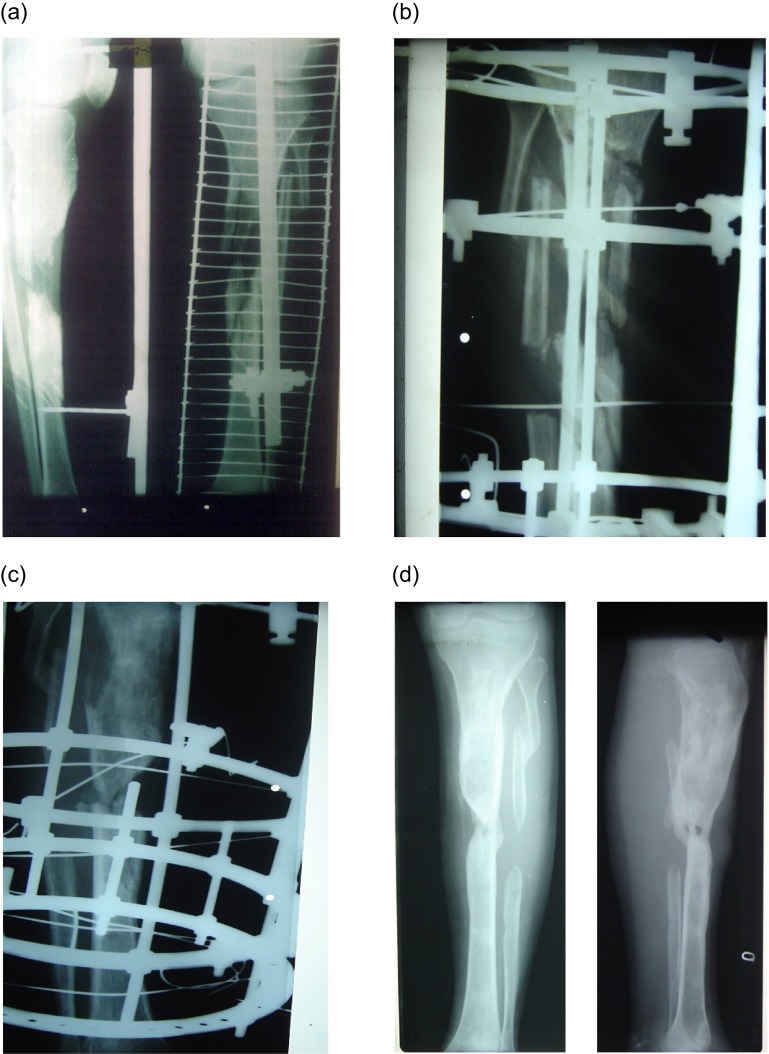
(a) AP and lateral pre-op X-rays showing Frank non-union with bone loss, (b) After applying IRF, corticotomy done at proximal tibia, (c) while undergoing distraction, (d) A/P and lateral views after union.
